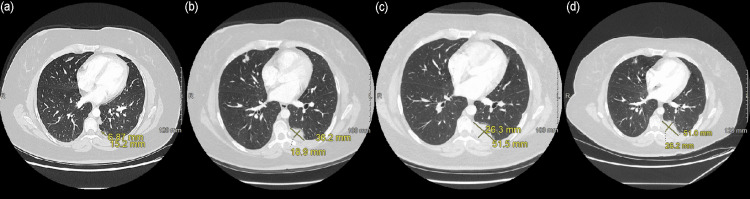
Chest CT demonstrating (a) left lower lobe pleural nodule post-cycle 12, (b) 4 months post-treatment, (c) post-cycle 3 resumption of TMZ/lapatinib and (d) post-radiation treatment changes to the pleural nodule.
